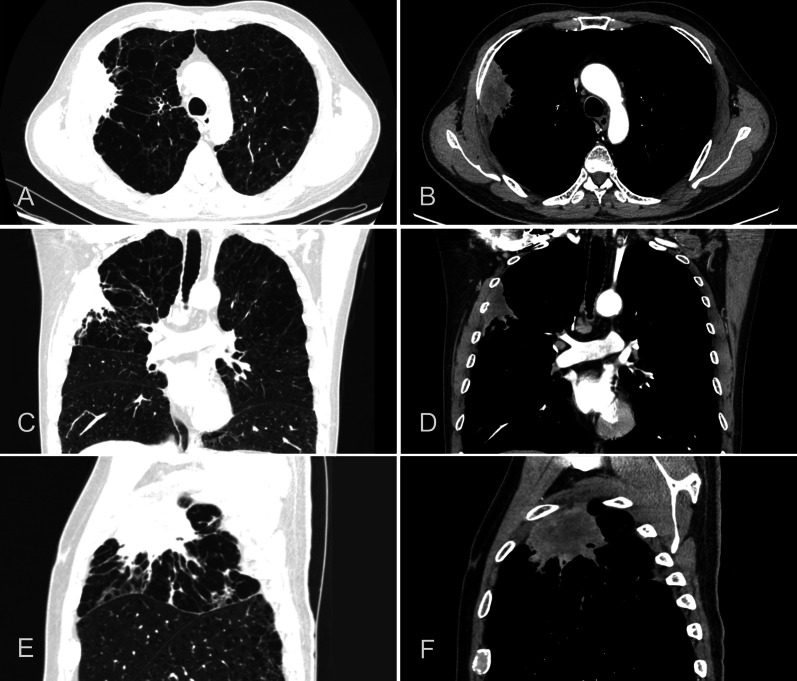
Preoperative enhanced computed tomography scan showing the right upper lobe mass. A Cross-section of the lung window; B cross-section of the mediastinal window; C coronal section of the lung window; D coronal section of the mediastinal window; E sagittal section of the lung window; F sagittal section of the mediastinal window
